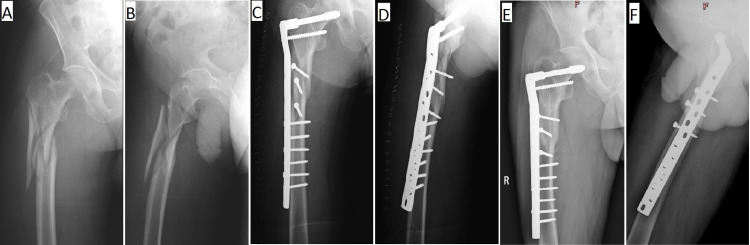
A 58-year-old male with a comminuted peritrochanteric fracture with subtrochanteric extension. A and B: Pre-operative X-rays. C and D: Immediate post-operative X-rays. E and F: One-year follow-up X-rays show a completely healed fracture.
